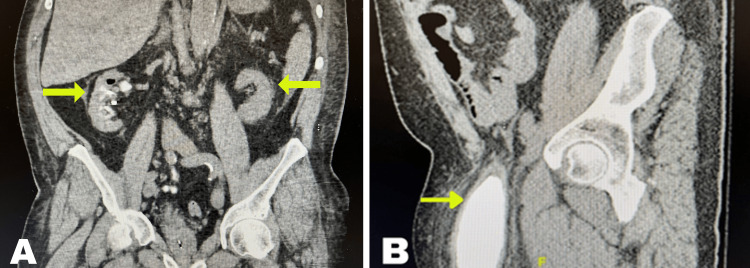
Abdominal CT scan showing: (A) bilateral atrophic kidney; (B) anterior aspect of the urinary bladder in the right inguinal hernia (coronal view) CT, computed tomography
